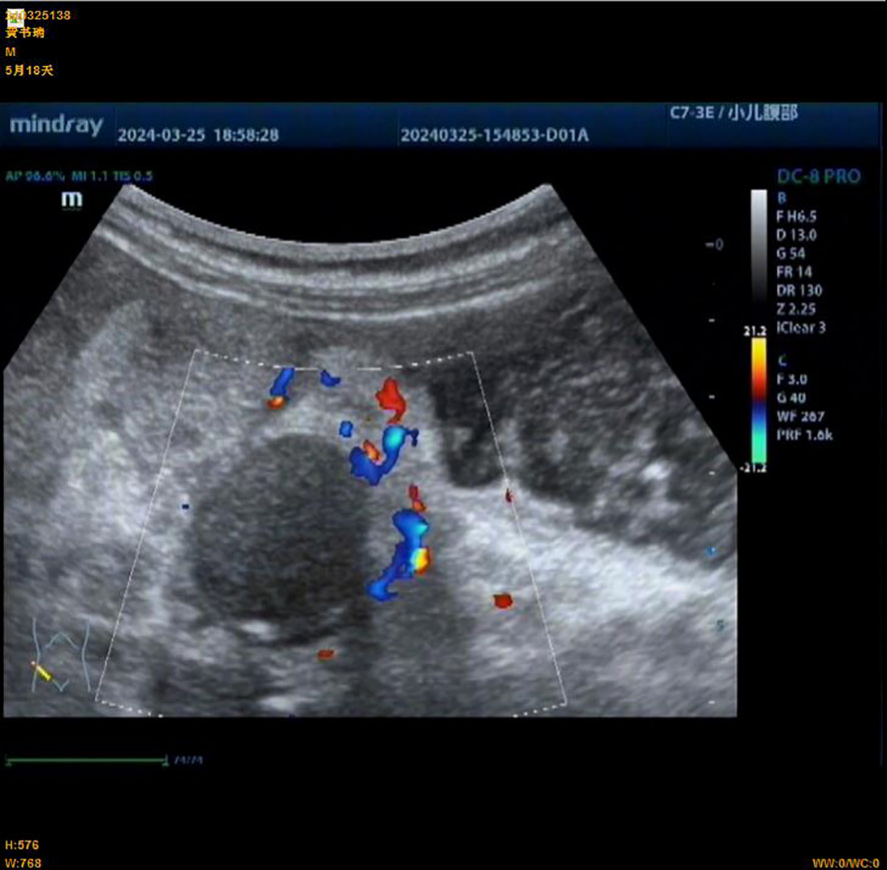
Preoperative abdominal ultrasound of an infant with congenital fibrosarcoma. An abnormal echo mass is seen in the right upper abdomen, with a range of approximately 31 × 19 mm, unclear boundaries, and uneven internal echoes.
